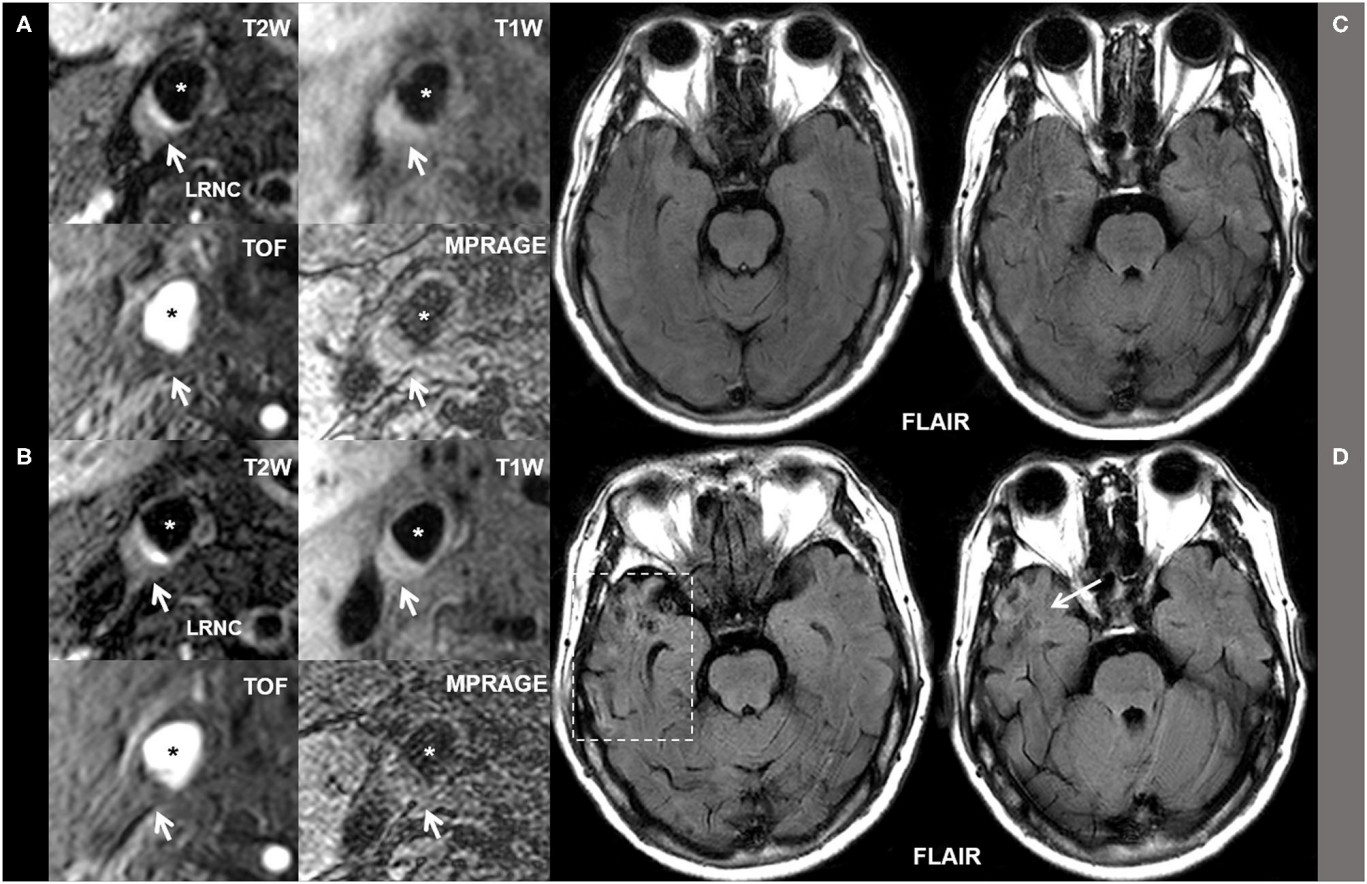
The baseline (A,C) and follow-up (B,D) MR images of a patient who had LRNC plaque in the right carotid with co-existing hypertension and H-Hcy (25 μmol/L). The LRNC (white arrows on T1W, T2W, TOF, and MP-RAGE images) in the right carotid artery and ipsilateral subsequent ischemic stroke (white arrows on Flair images) in the temporal lobe had progressed after 1-year follow-up. * Marks lumen of common carotid artery.
